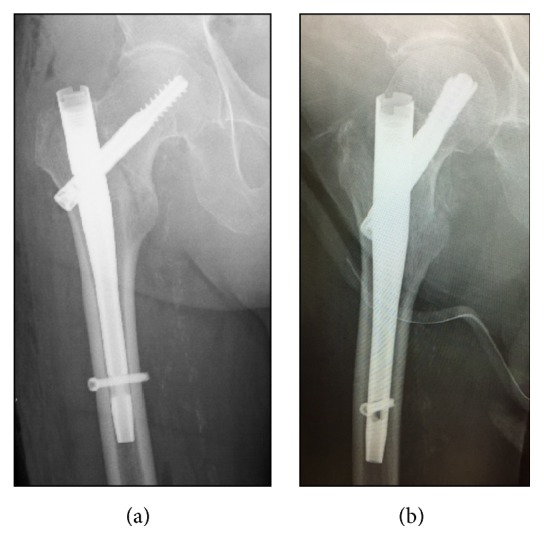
Postoperative anteroposterior (a) and lateral (b) radiographs of the pelvis demonstrating satisfactory short femoral nail fixation of the intertrochanteric hip fracture.
